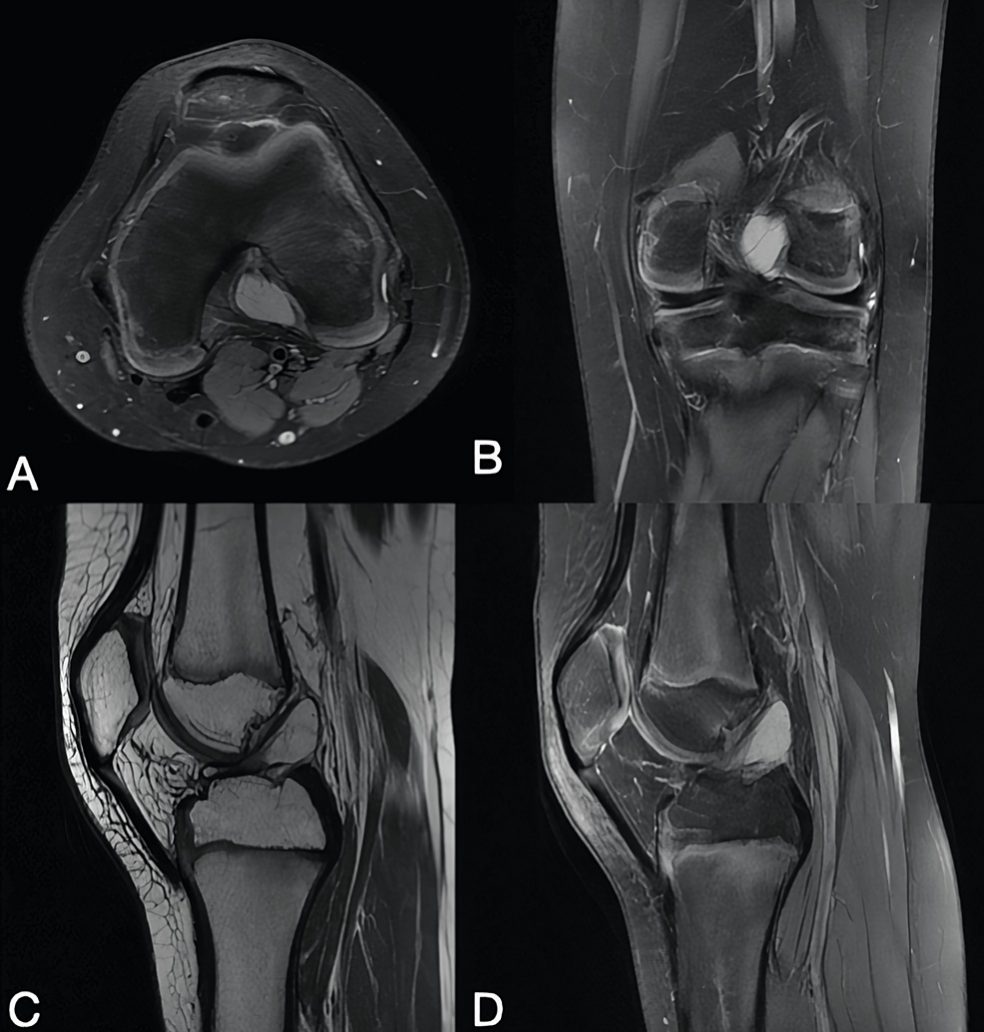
A: Preoperative left knee MRI in axial, B: Coronal, C and D: Sagittal planes " C, D". revealed a well-defined lesion with fluid signal intensity in the intercondylar notch, measuring 2.1 x1.7 x1.5 mm, which was eccentric to compression and closely related to the anterior cruciate ligament. A corticated bone fragment was noted within Hoffa's fat pad.
